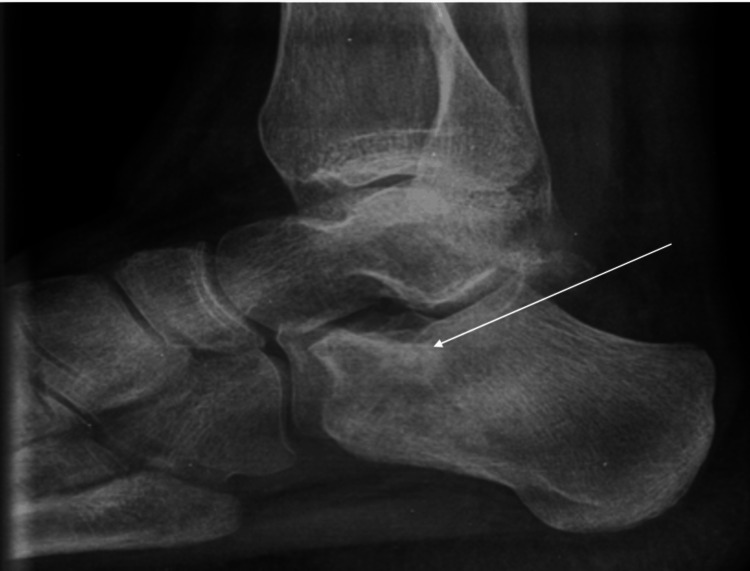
Plain X-ray (sagittal view) of calcaneus The white arrow depicts a small, central periosteal core reaction with cortical thickening (nidus) on the anterior and superior aspect of the calcaneus. This lesion is visible as a radiolucent area.
